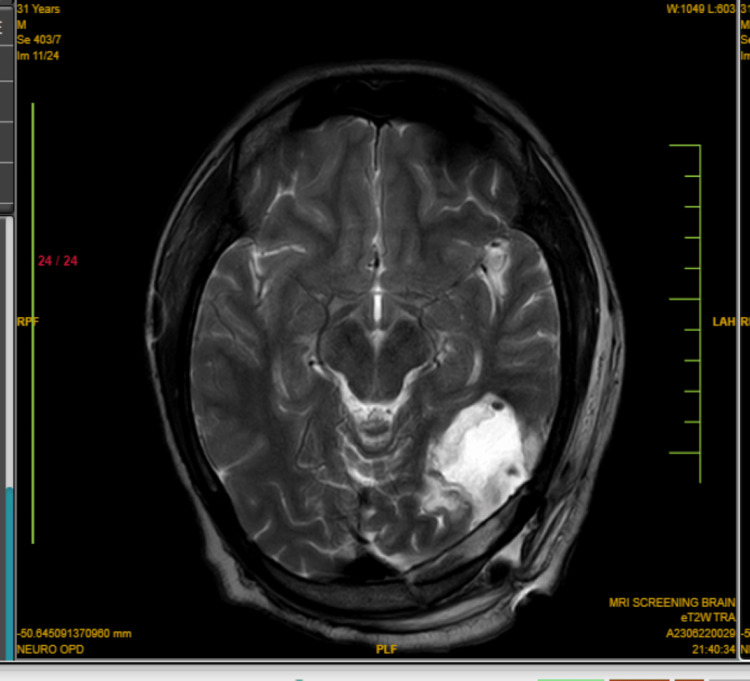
MRI brain shows multiple areas of postoperative calvarial defect in the left temporo-occipital region with heterogeneously T2/FLAIR hyperintense collection noted in the subdural space. FLAIR: Fluid-attenuated inversion recovery
